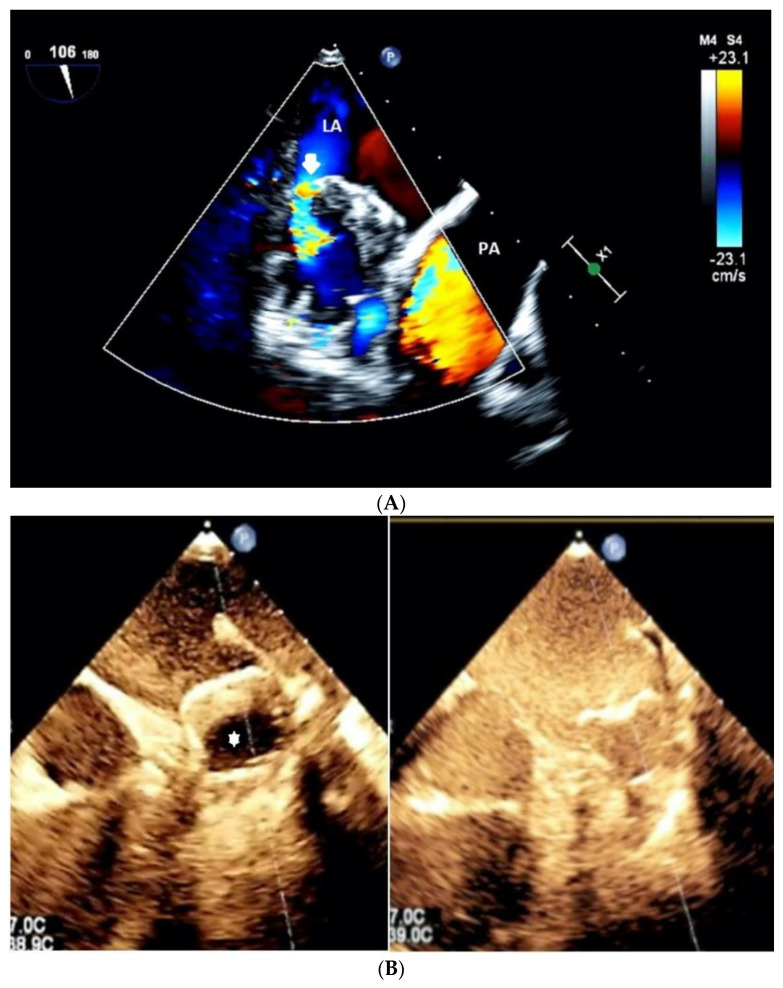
(A,B) A Color Doppler interrogation (A) and Ultrasound-enhanced imaging using SonoVue injection of the LAA device. Arrow signifies the inflowing blood flow inside the LAA through the PDL, explaining the pathophysiology of type C lesion. In (B), the echo-free area (*) of the device was filled completely after implication of contrast study.
